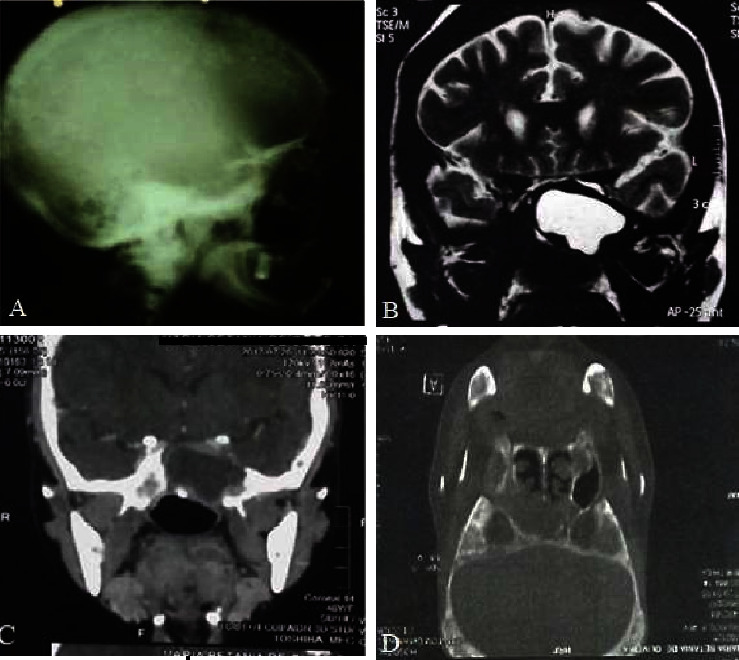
(A) Skull X-ray: enlargement of the sella turcica. (B) Skull MRI in T2 and (C) skull MRI in T1: presence of a 3.1 cm lesion invading the pituitary fossa. (D) MRI in T1: infiltrative lesion compromising the bony segments of the skull and face.
